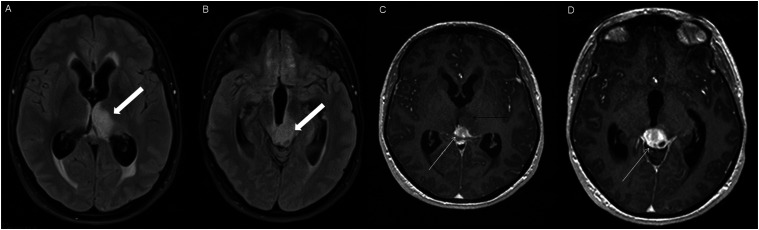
Axial FLAIR (A-B) and post-contrast T1 weighted images (C-D), show a FLAIR hyperintense mass involving the left thalamus and quadrigeminal plate (A-B, thick white arrows), with mixed non-enhancing (C, thin black arrow) and nodular-enhancement components (C-D, thin white arrows). There is mass effect and obstruction of the third ventricle with obstructive hydrocephalus and transependymal CSF flow.
